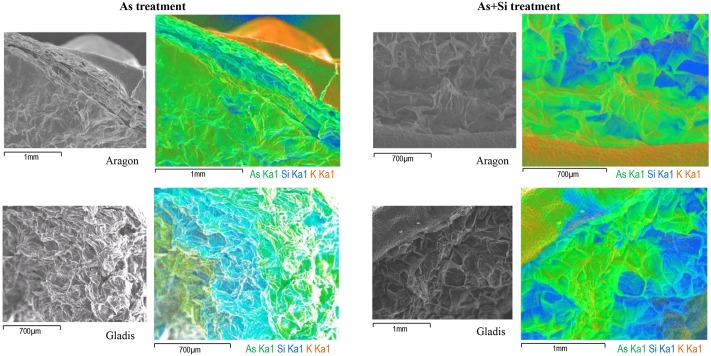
SEM/EDX fruit multi-element distribution maps. Fruit Secondary Electrons (SE) image, As (Kα1), Si (Kα1), K (Kα1) emission X-ray multi-element map, for Aragon (Top) and Gladis (bottom) under As (Left) and As+Si (Right) treatments. The map colors code is: green for As, blue for Si, and orange for K. In SE images black bar at bottom left indicates cross section size.
